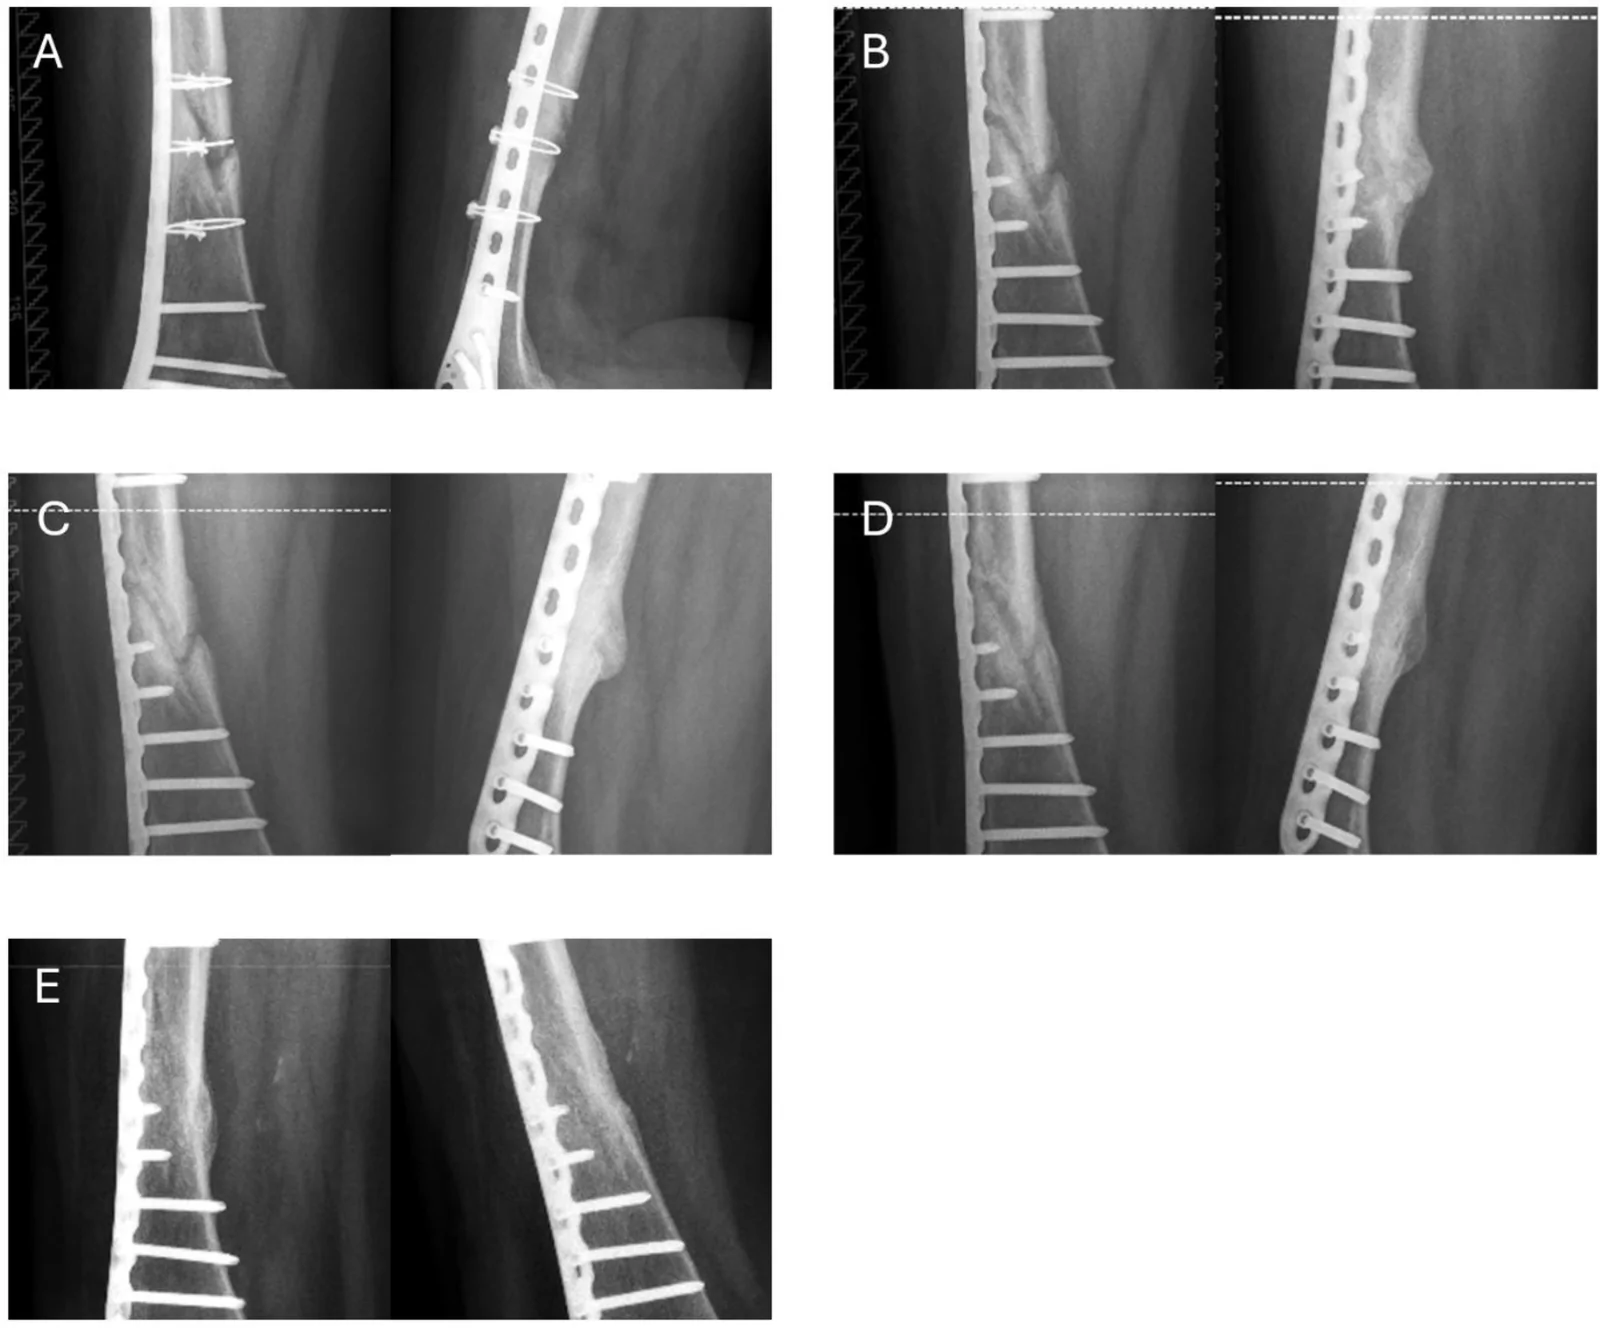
Example 2 of a case with a femur non-union during a 10-year follow-up. (A) Preoperative X-ray (B) Postoperative X-ray after 6 months. (C) Postoperative X-ray after 12 months. (D) Postoperative X-ray after 24 months with full osseus consolidation with a LSS of 3. (E) Postoperative X-ray after ten years with fill osseus consolidation (LSS 4)
